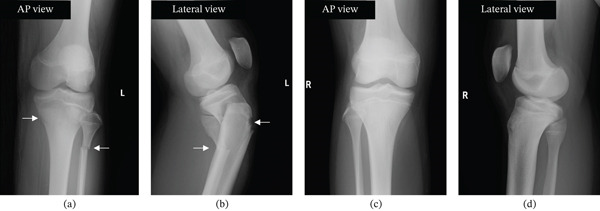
Initial radiographs of both knees. (a) Plain x‐ray image of the left knee joint at the time of the initial visit to our department. Anteroposterior (AP) view of the left knee showing a proximal tibial physeal fracture (arrow) and a fibular fracture (arrow). (b) Plain x‐ray image of the left knee joint at the time of the initial visit to our department. Lateral view of the left knee demonstrating displacement of the proximal tibial physeal fragment (arrow) and a fibular fracture (arrow). (c, d) Plain x‐ray image of the right knee joint at the initial visit to our department. There are no findings suggestive of rickets, such as cupping or flaring: (c) AP view and (d) lateral view.
